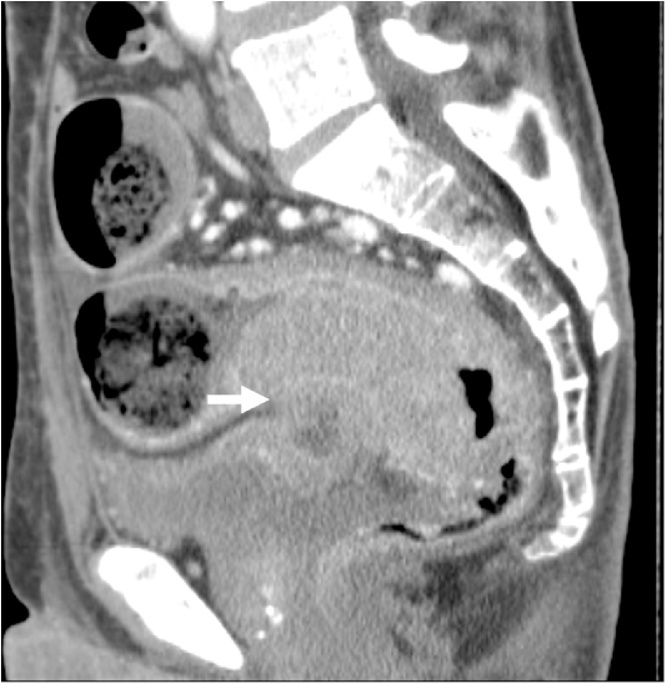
Abdominal CT revealed that the wall thickness of the rectum showed extensive invasion of the bladder wall (arrow). CT, computed tomography.
